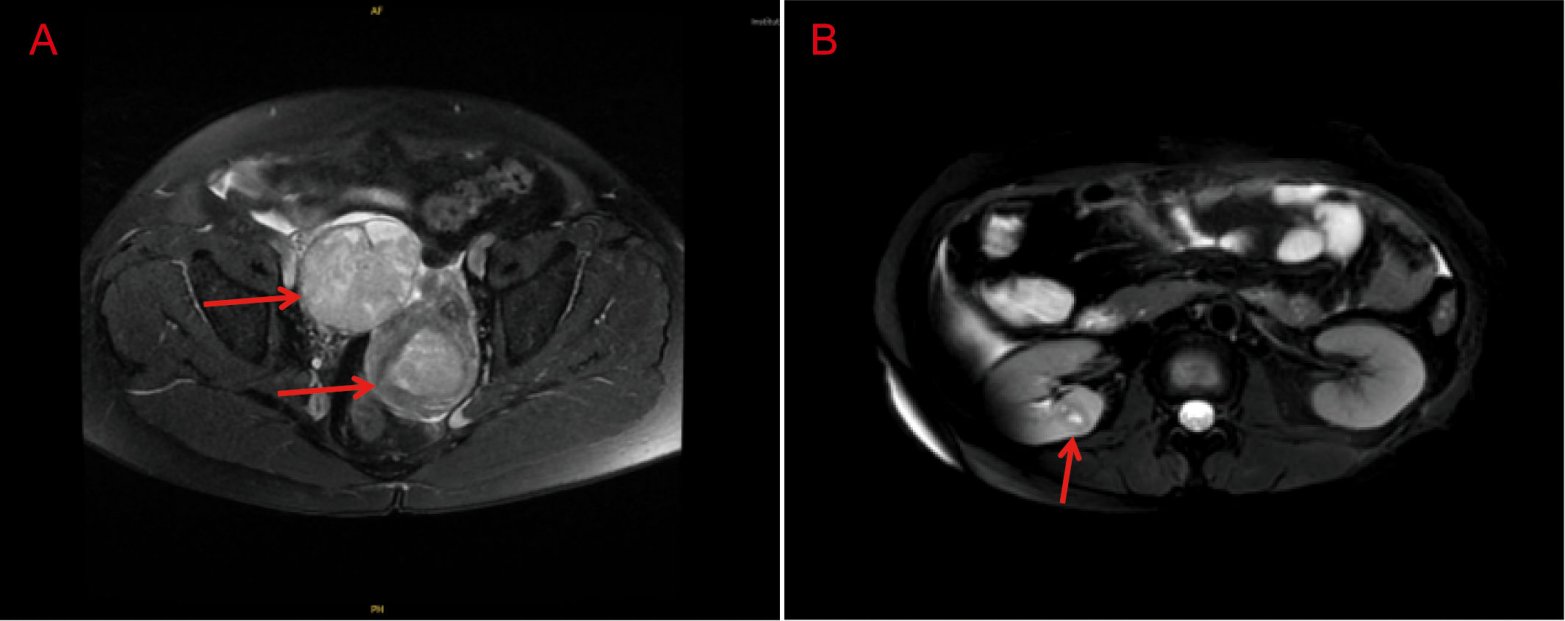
Imaging findings of the patient. (A) MRI scan showed uterine cavity mass (4.3×3.0×3.7cm) with T2 hyperintensity and heterogeneous enhancement; right adnexal irregular solid mass (8.2×6.4×6.5cm) with cystic components and peripheral enhancement, no pelvic lymphadenopathy or peritoneal nodules. (B) Right renal parenchymal mass with clear borders, T1 hypointensity, T2 isointensity, and mild heterogeneous enhancement.
